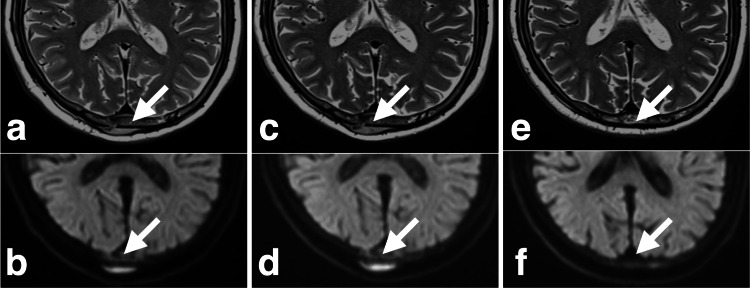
Time course of the occipital lesion on MRI T2WI and DWI (white arrows) pre-CKR: (a) T2WI, (b) DWI. Day 5 after starting CKR: (c) T2WI, (d) DWI. Day 44 after starting CKR: (e) T2WI, (f) DWI. MRI: magnetic resonance imaging, T2WI: T2-weighted image, DWI: diffusion-weighted image, CKR: CyberKnife radiotherapy
